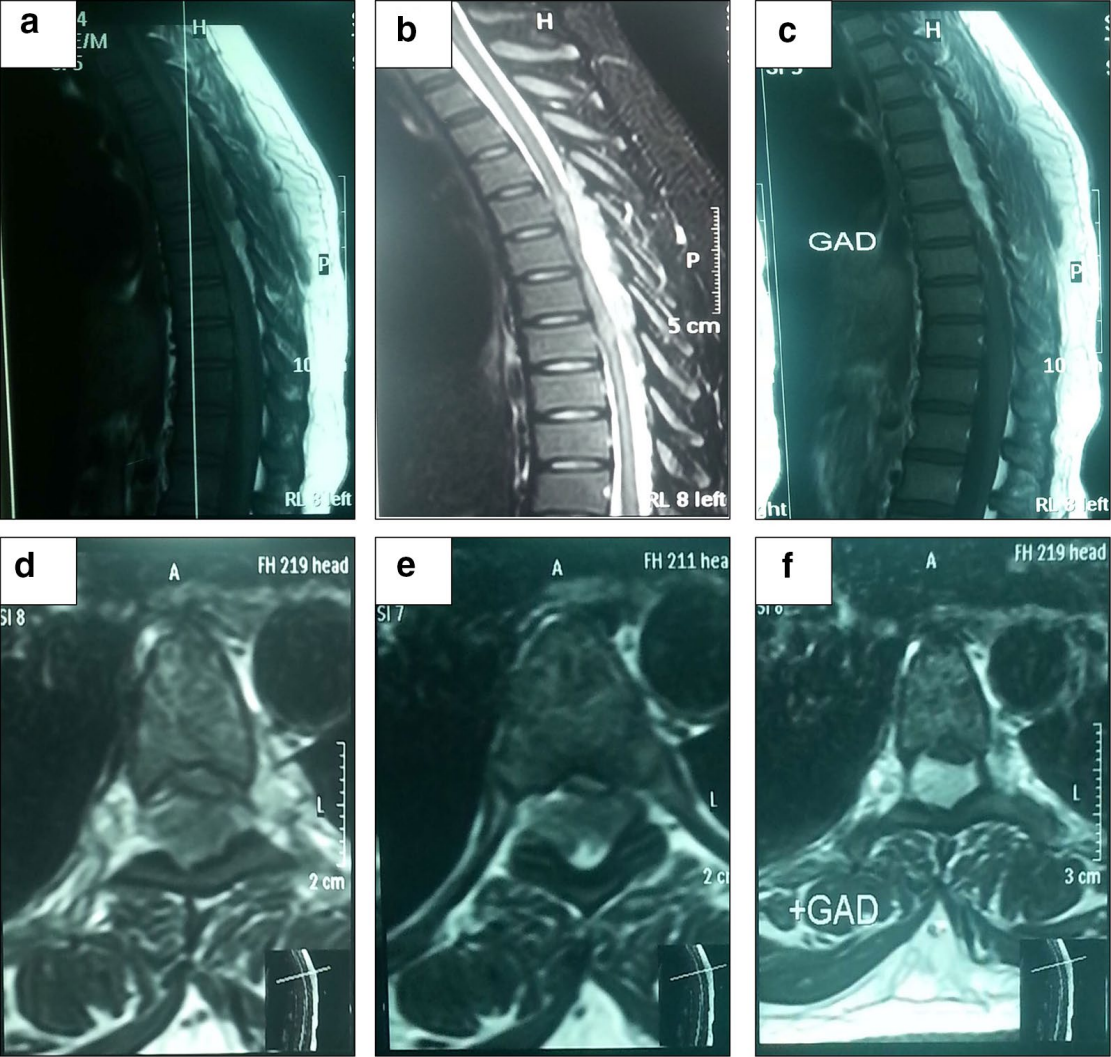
a Non-contrast T1-weighted sagittal MRI of the mid thoracic spine showing a very inhomogeneous mass, extending from D5 to D8. The tumor shows a large component hypointense to fat. b T2-weighted sagittal MRI showing the mass as hyperintense relative to spinal cord and nearly isointense with normal fat and myelopathic changes in the cord. c Post-contrast T1-weighted sagittal MRI showing diffuse, slightly inhomogeneous enhancement of the mass. Non contrast T1-weighted axial MRI (d) T2-weighted axial MRI (e) and post-contrast T1-weighted axial MRI (f) the mass along the posterior epidural spinal canal of the dorasl spine, compressing and displacing the spinal cord anteriorly
